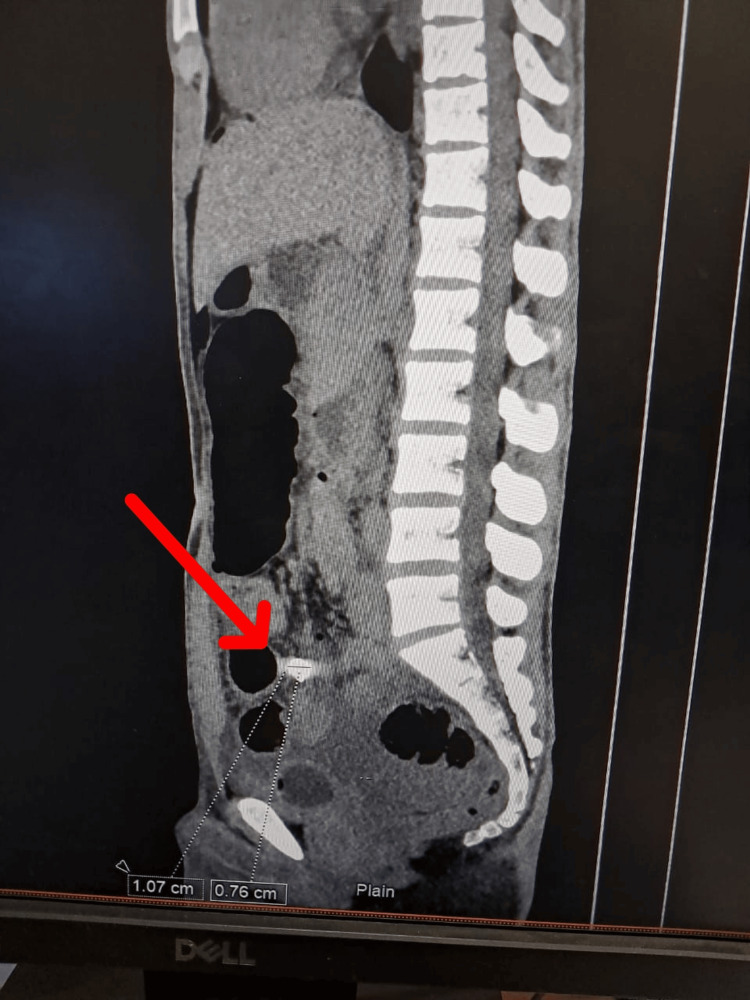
NCCT images localizing the foreign body (arrow). NCCT: non-contrast computed tomography
